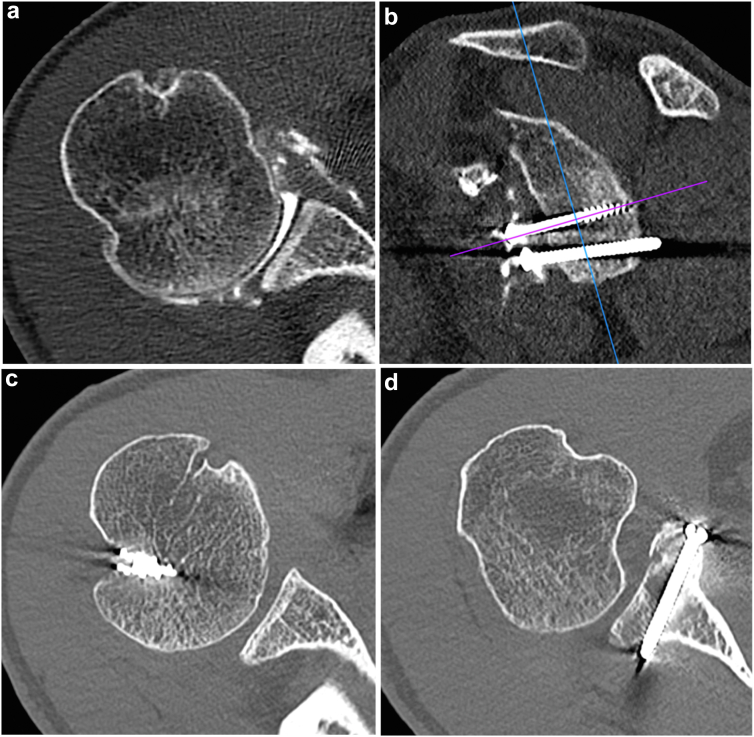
Pre-operative CT scan (a and b) showing bone block osteolysis and moderate Hill-Sachs lesion (a and b). Post-operative CT scan at 4 months follow-up showing bone block consolidation (c and d). *CT*, computed tomography.
